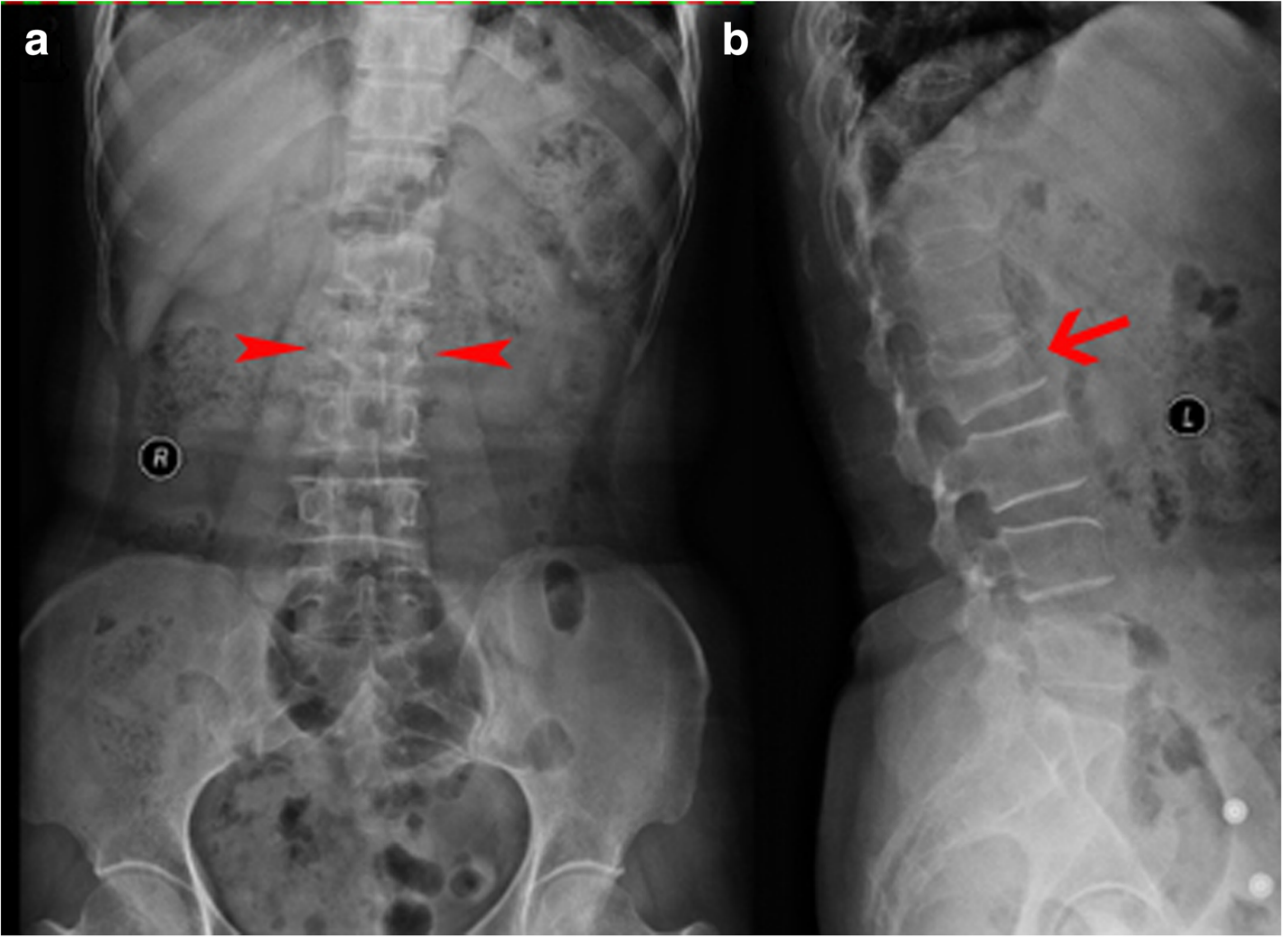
Typical radiographic views. a, b Preoperative anteroposterior and lateral radiographs showing a compression fracture of the second lumbar vertebra
